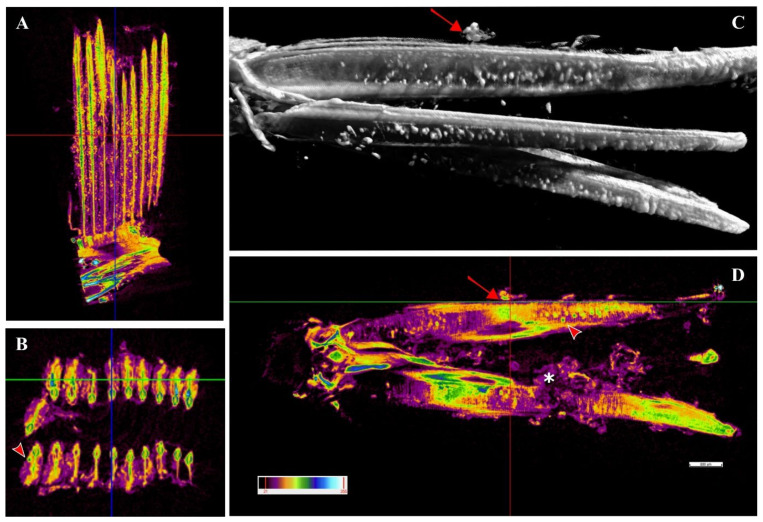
X-ray micro-computed tomography of greater amberjack infected gills (September 2018). (A,B,D) Two-dimensional cross sections of a partial gill arch seen along three different axes. Density of tissue is displayed with a range of colours from black to white. Denser structures are in white, blue and green. (C) Three-dimensional volume rendering of three filaments. Cysts are seen as yellow spherical structures between the secondary lamellae and when the section cuts through them they display a green (denser) colour (arrowheads). Proliferative epithelial tissue is also seen outside and between the filaments (asterisk) and, when density-inspected, the presence of cysts is revealed (arrows).
